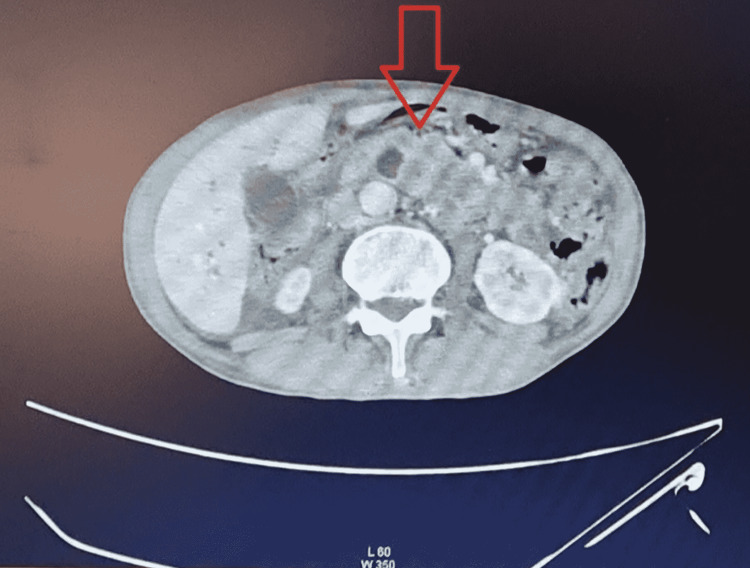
Mass on the pancreatic head in contrast-enhanced abdominal CT scan. CT: computed tomography
